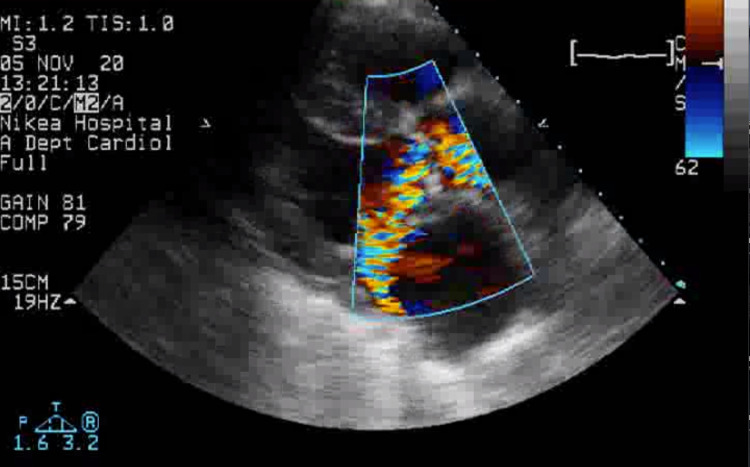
Doppler mode in TTE of the patient’s native aortic valve. TTE: transthoracic echocardiography)
